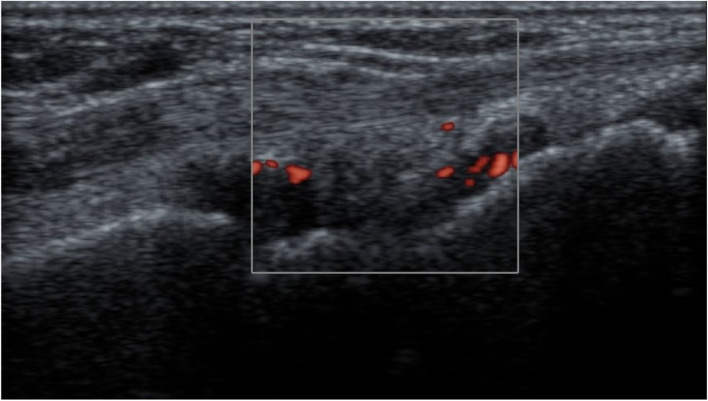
PDUS dorsal longitudinal scan showing grade II Doppler activity in wrist joint
